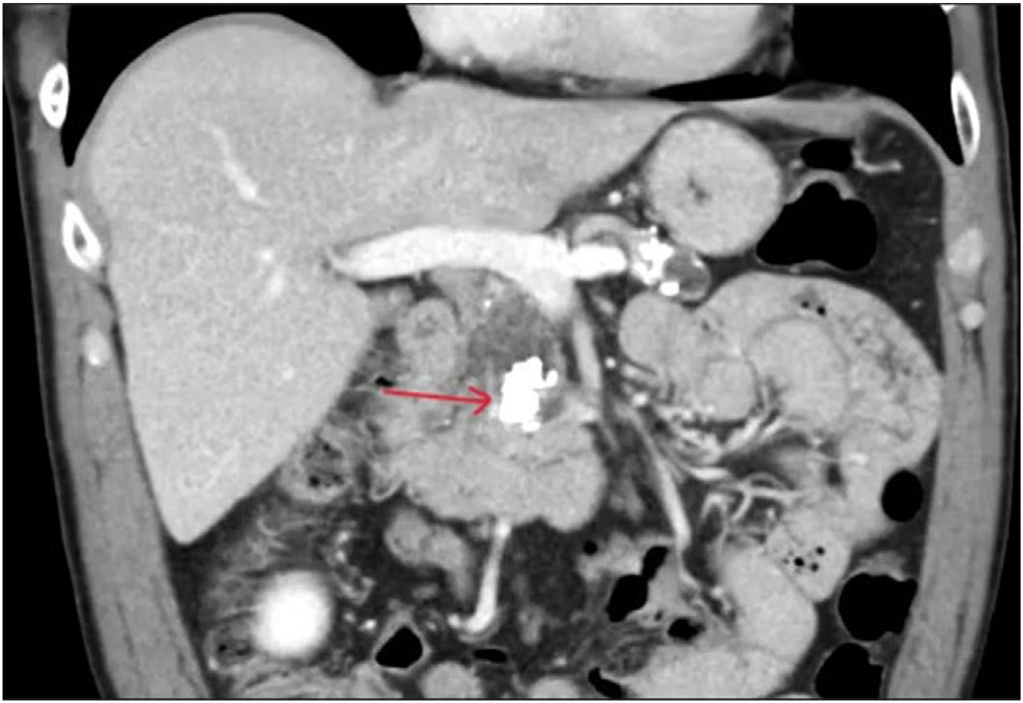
CT image demonstrating a large burden of calcified obstructive pancreatic stones impacted above the papilla (*red arrow*).
